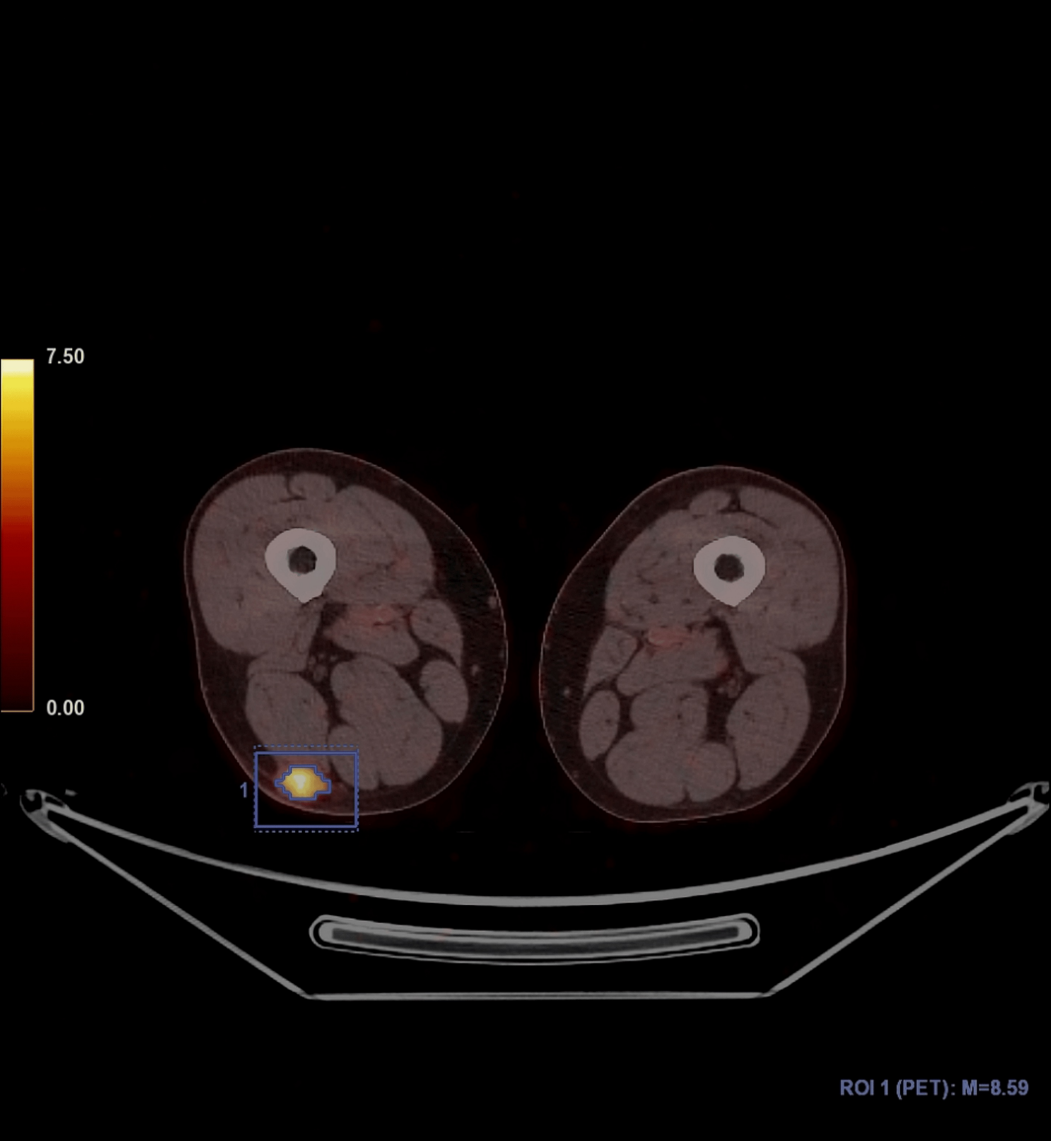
Mass lesion on PET-CT Soft tissue mass lesion located at right thigh displaying high FDG uptake (within square) (PET-CT).
